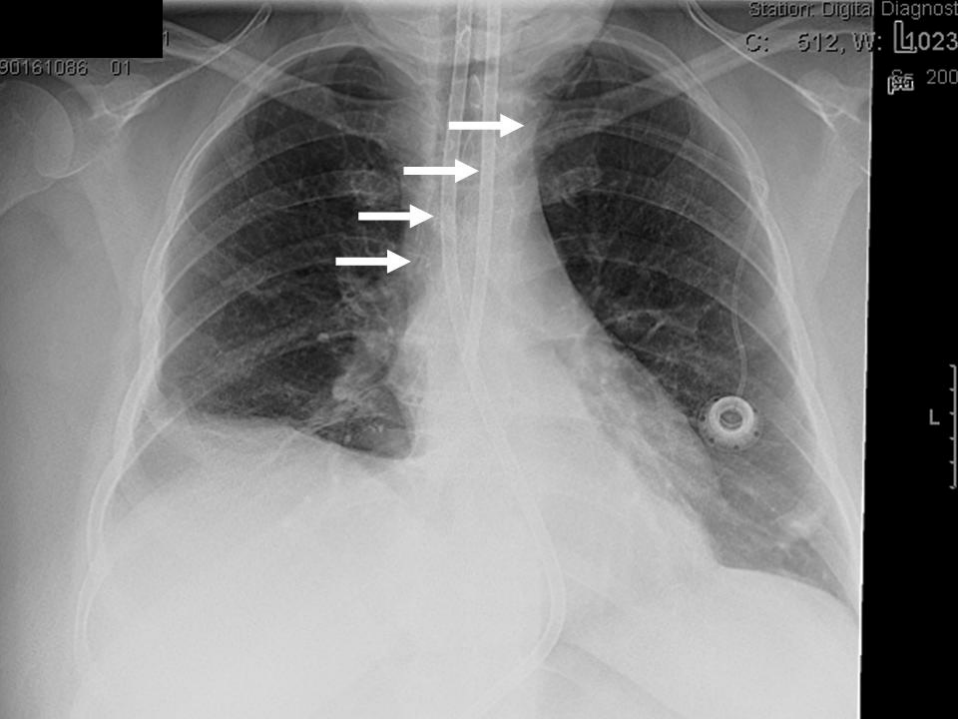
Radiograph of the chest following implantation of the Port-A-Cath catheter. The tip of the catheter was advanced to the distal part of the superior vena cava from the left subclavian vein (white arrows).
